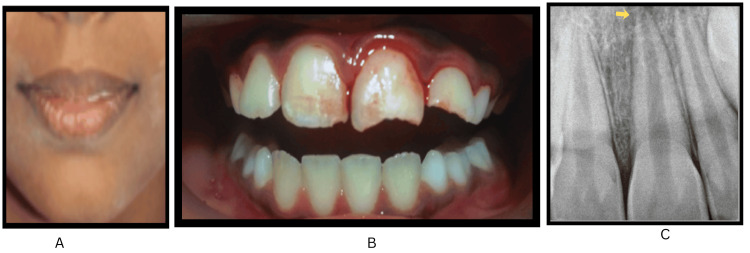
Clinical and radiographic images of the replanted maxillary left central incisor with associated crown fractures. (A and B) Intraoral and extraoral clinical photograph showing the replanted avulsed maxillary left central incisor (tooth #21) with associated uncomplicated crown fractures in teeth #11, 21, and 22. (C) Immediate intraoral periapical radiograph confirming the proper positioning of the replanted tooth #21, which presents with an open apex consistent with Cvek stage 4 root development (marked by arrow).
